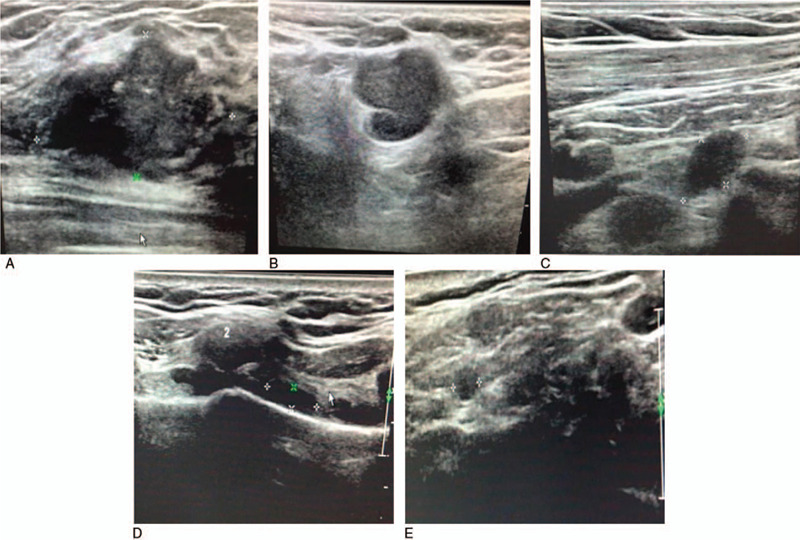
Imaging of right breast mass and right draining lymph node by ultrasound. (A) Right breast mass; (B) Right subaxillary lymph node; (C) Right subclavian lymph node; (D) Right internal mammary lymph node; (E) Right supraclavicular lymph node.
